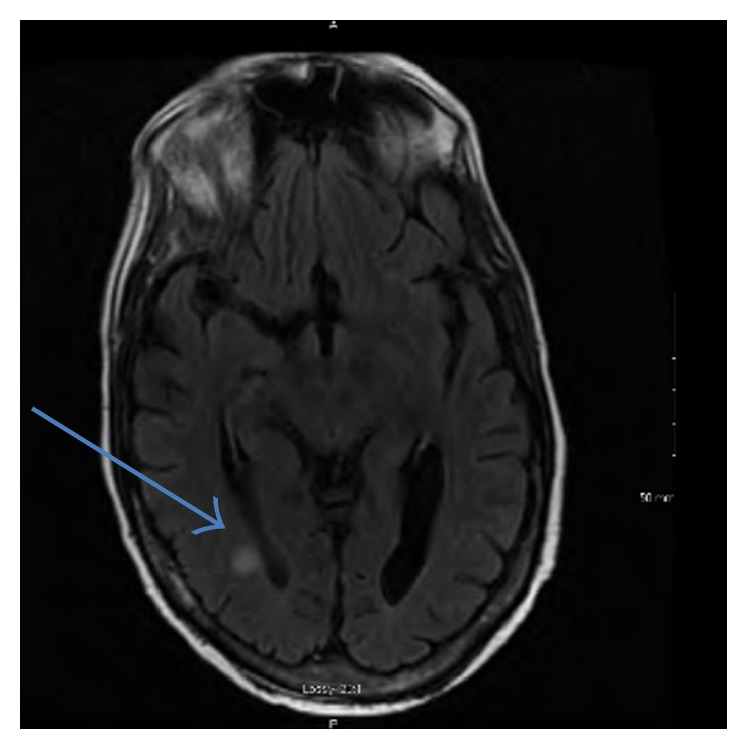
MRI of the brain. Multiple ring-enhancing lesions with associated diffusion restriction and T2/FLAIR hyperintensity are present throughout the supratentorial white matter.
